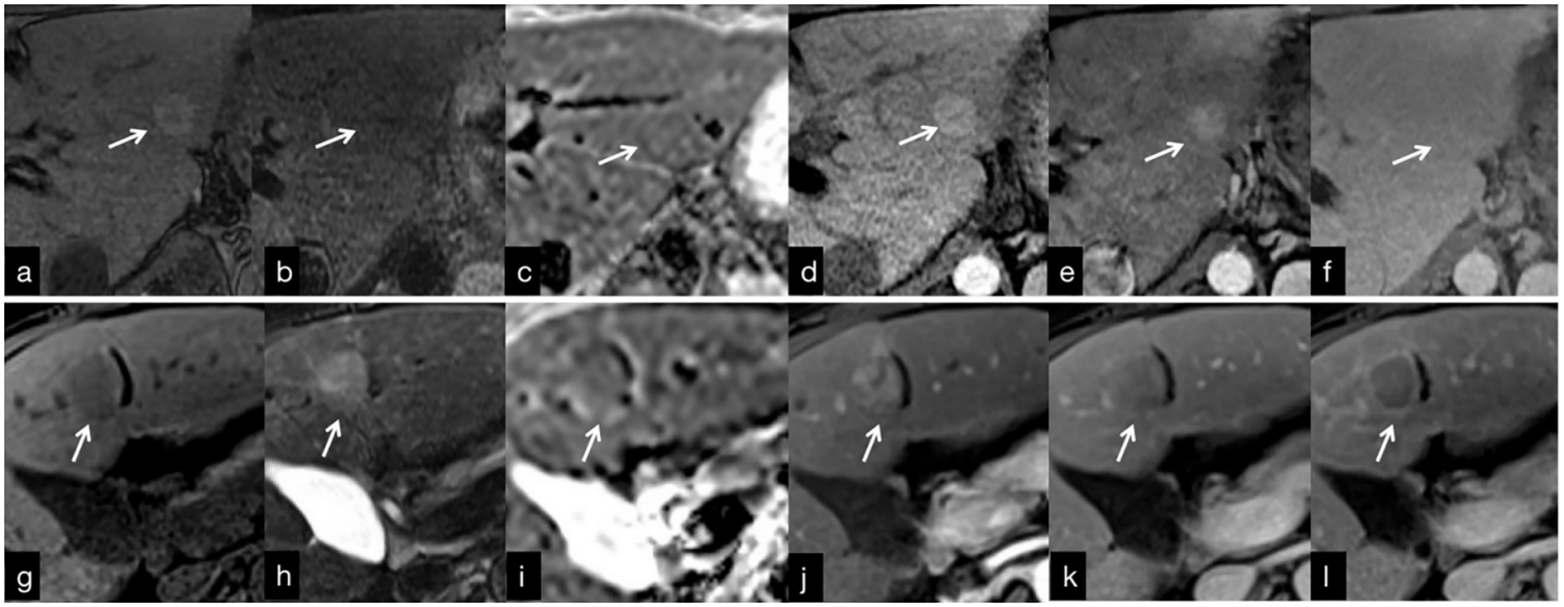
(A–F) MRI findings in a 30-year-old man with Budd-Chiari syndrome. (A) There was an RN in segment 2 (arrow) that is hyperintense on T1WI, with a hypointense central scar. (B) T2WI shows a homogeneous slightly hypointense lesion. (C) On the ADC map, the lesion is slightly hyperintense. (D) The arterial phase image shows homogeneous enhancement that persists in the venous (E) and delayed (F) phases. g-f: MRI findings in a 61-year-old woman with hepatocellular carcinoma. There is a s-HCC in segment 4 (arrow). (G) T1WI shows a hypointense lesion that is slightly hyperintense on T2WI (H). (I) ADC map shows slightly hypointense lesion. The lesion is hypervascular in the arterial phase (J), with washout in the venous (K) and delayed (L) phases.
